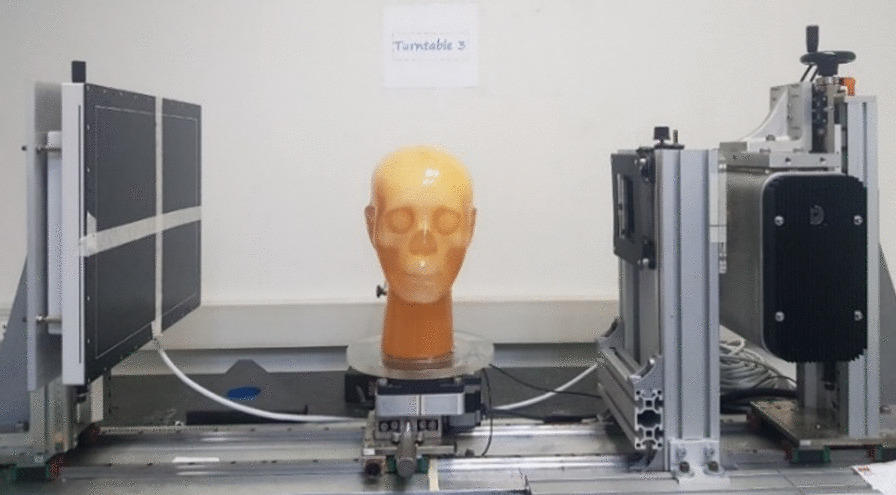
The CBCT scanner prototype on a benchtop system
